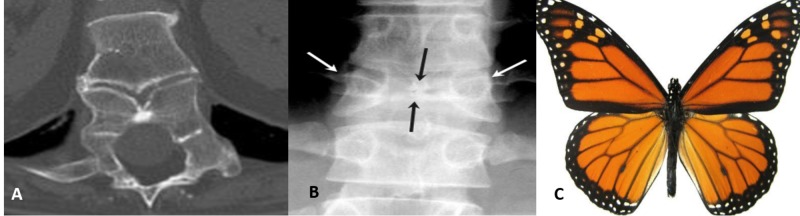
Butterfly vertebrae X-ray and computed tomography scan showing butterfly vertebrae.
